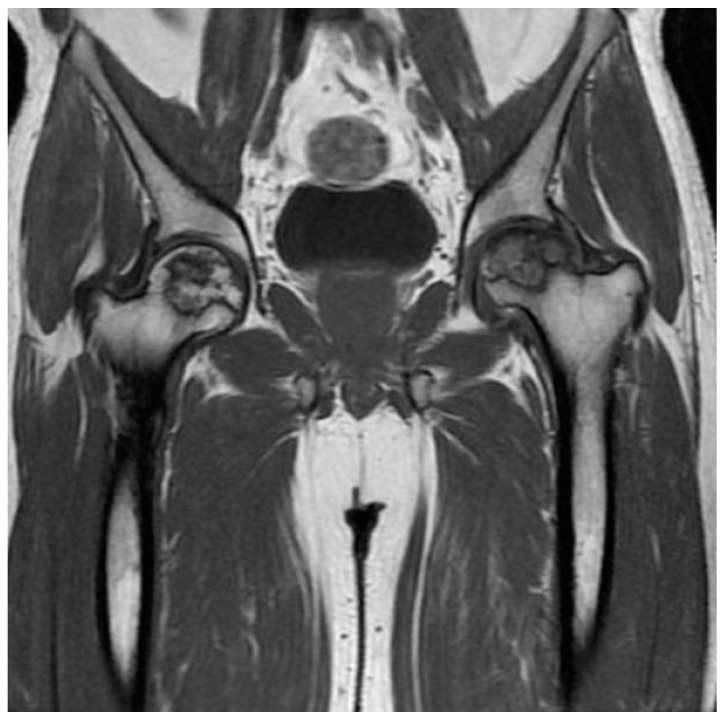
T1-weighted image of bilateral AVN of the femoral head.
